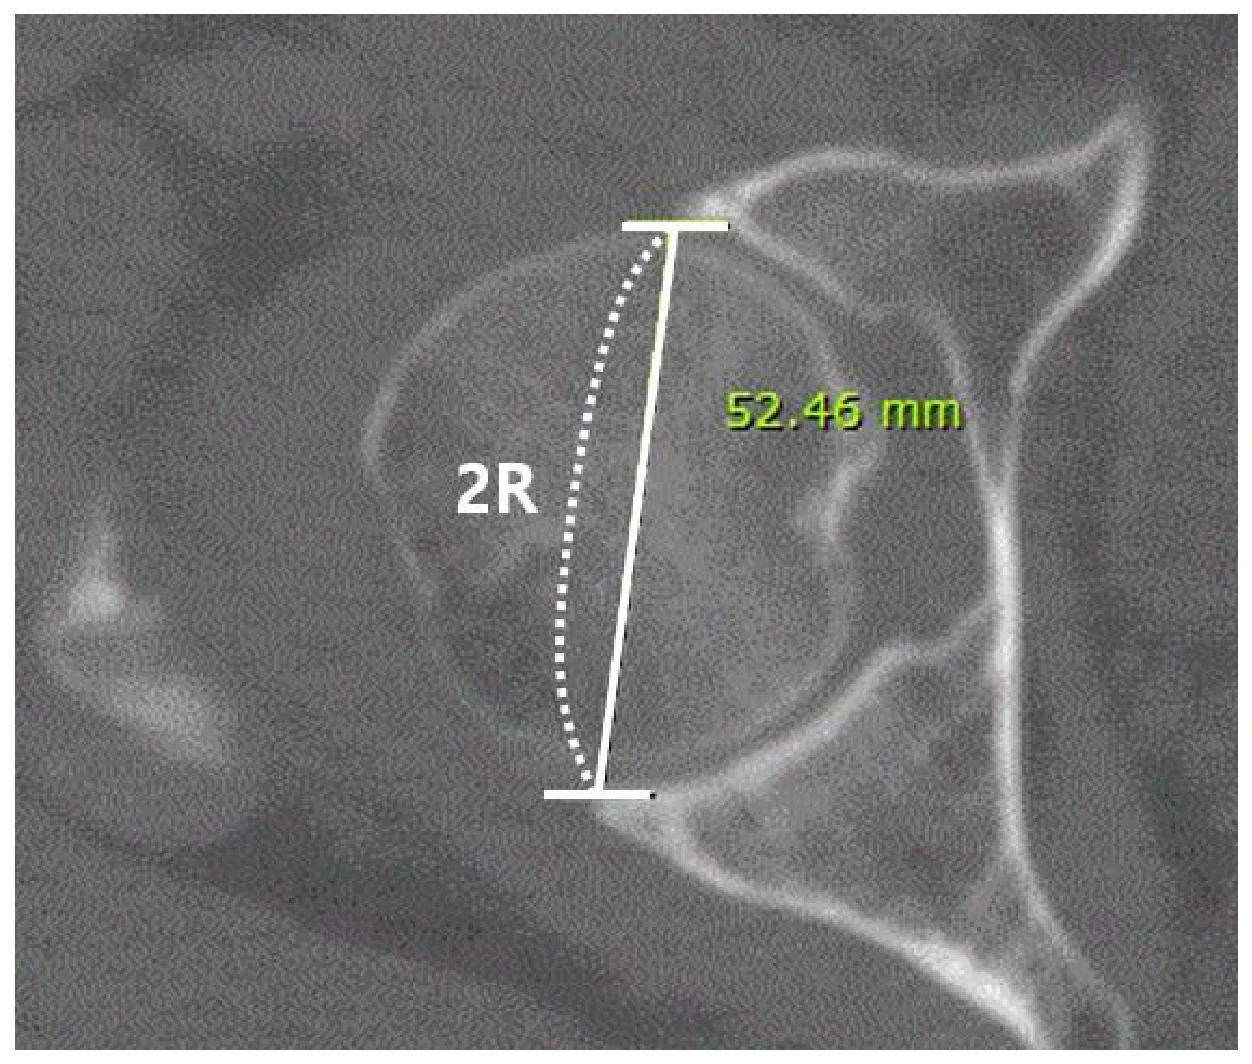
The distance between the anterior and posterior poles of the acetabulum measured on a mid-axial CT image.
